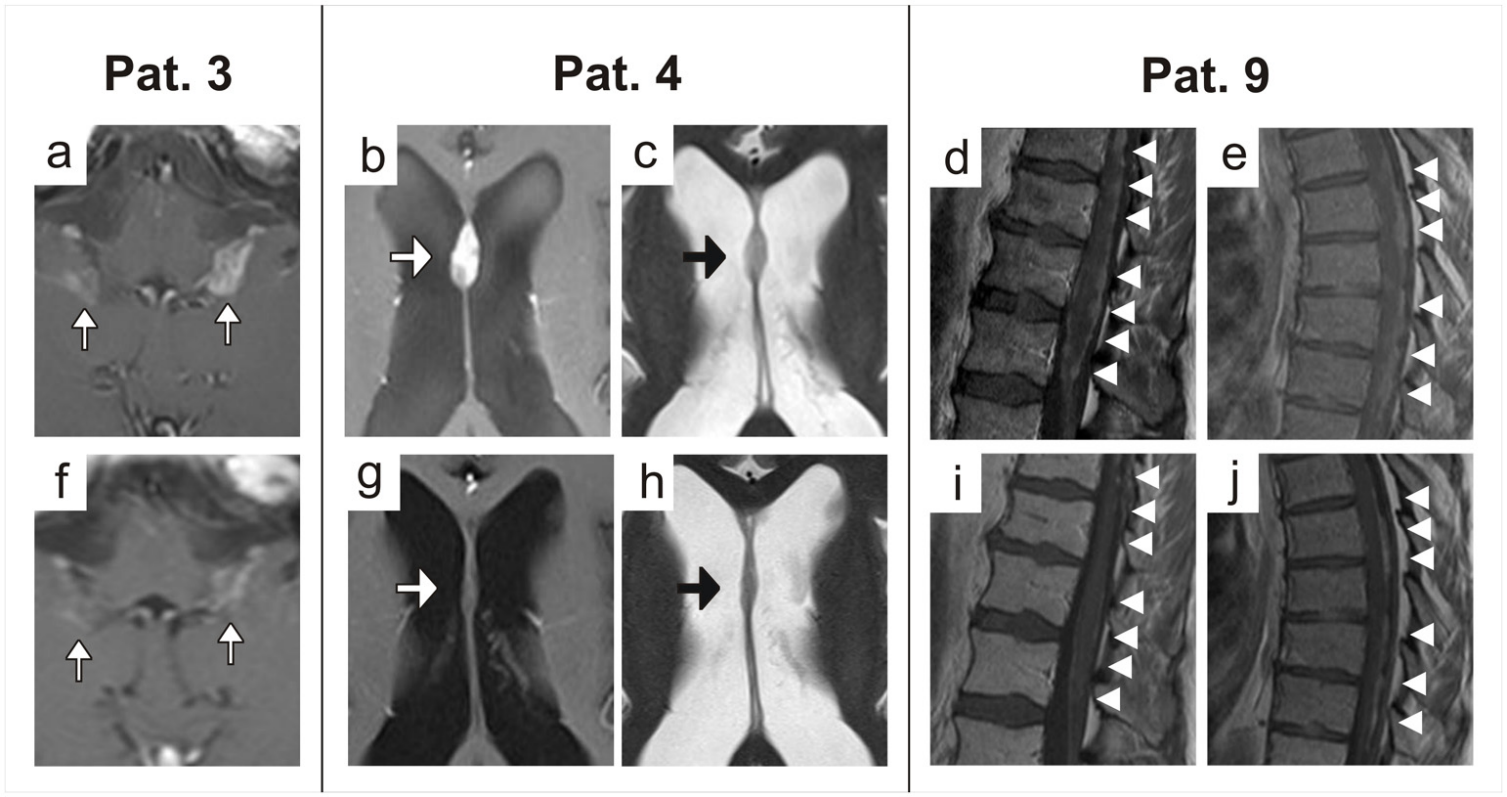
MRI scans before (a-e) and under (f-j) therapy. a, f: Reduced leptomeningeal enhancement (white arrows) after 8 weeks of therapy with bevacizumab and lomustine in patient 3. b, g: Regression of leptomeningeal contrast-enhancing nodule (white arrow) on the septum pellucidum on T1-weighted images after eight weeks of therapy with bevacizumab and temozolomide in patient 4. c, h: This regression (black arrow) in patient 3 was also visible on T2-weighted images, which makes pure pseudoresponse unlikely. d, i: Regression of leptomeningeal contrast-enhancing nodules (white arrowheads) on the surface of the medullar conus and the lumbar nerve roots on T1 weighted images (Th10-L2) in patient 9 before and after radiotherapy plus eight weeks of therapy with bevacizumab and lomustine. e, j: This regression of contrast-enhancement (white arrowheads) in patient 9 was also apparent in the thoracic spine (Th5-Th9) which was not treated with radiotherapy.
